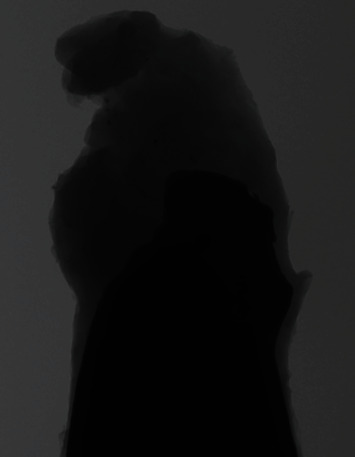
Micro-computerised tomography (CT) radiograph of the soft tissue mass and apex of tooth #21.
